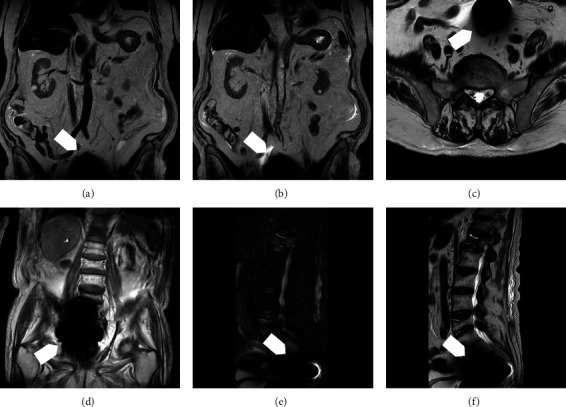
(a) Coronal fast-spin echo T1- and (b) T2-weighted images from an unenhanced abdominal MR study showing extensive paramagnetic “blooming” artifact at the lower end (arrow), corresponding to the site of the tip tungsten ballast. Axial T2- (c), coronal T1- (d), sagittal fat-suppressed (e), and T2- (f) weighted images performed to assess osteoporotic vertebral collapses show paramagnetic artifact (arrows) partially obscuring the anterior aspect of the sacrum.
